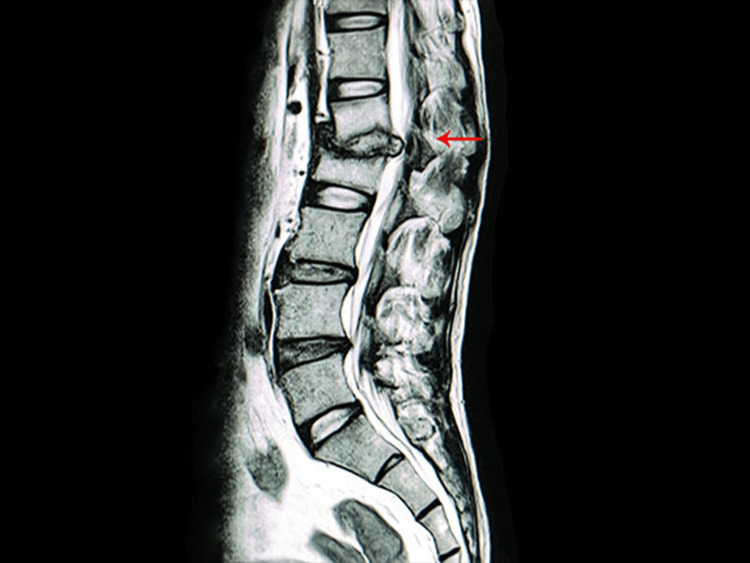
An MRI depicting tuberculosis of the spine T2 weighted sagittal MRI cut of the lumbosacral spine, red arrow depicting discal and paradiscal involvement of the L1-L2 disc and endplates of L1 and L2 with compression of posterior neural structures most probably due to infective etiology due to tuberculosis of the spine (Pott's spine) This image is from the authors' institution.
